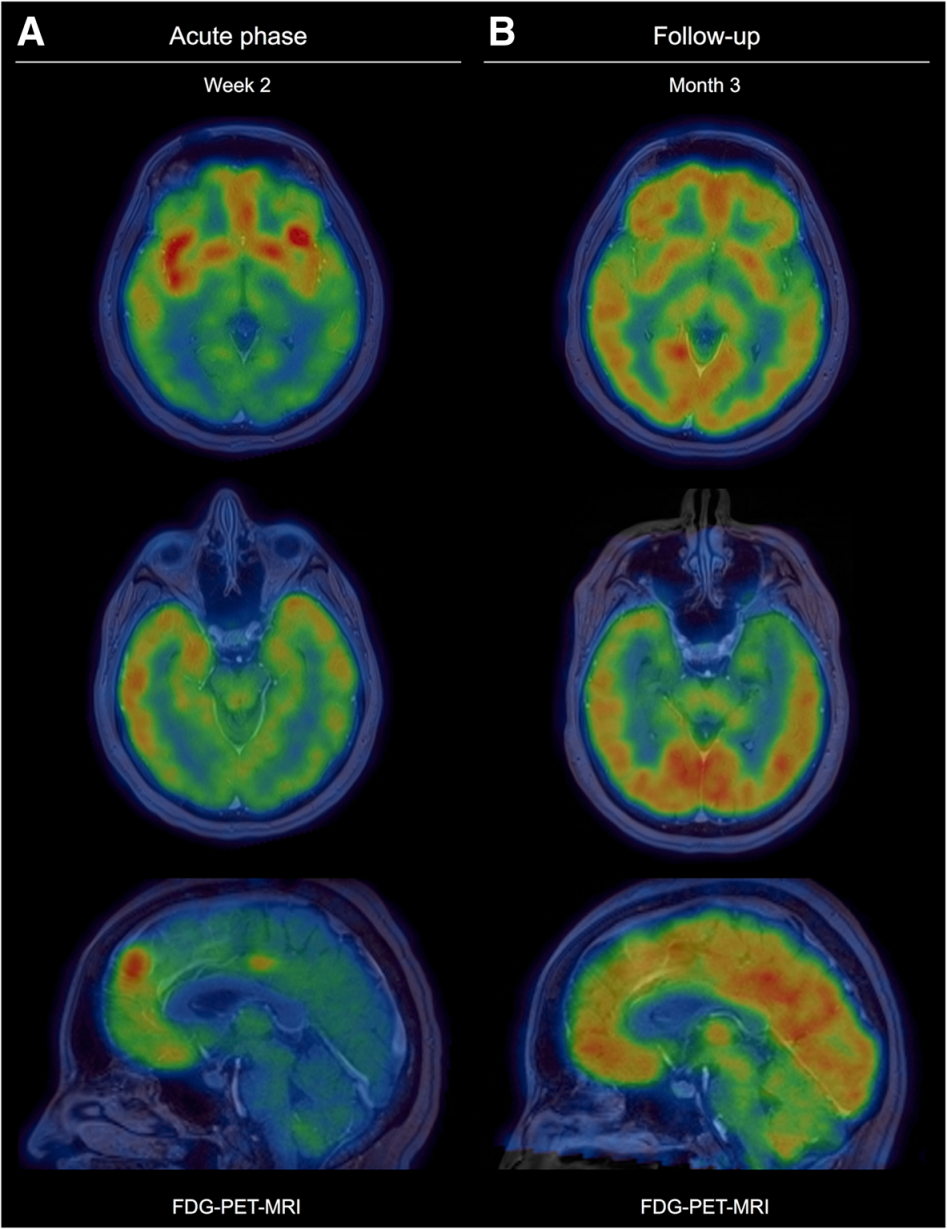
Evolution of glucose metabolism over time. a F^18^-FDG-PET during acute phase of disease (2 weeks after onset) shows bi-insular and bi-frontal glucose hypermetabolism as well as glucose hypometabolism occipital and partially in the parietal lobe. b After 3 months there is a marked bi-insular and bi-hippocampal hypometabolism, whereas prefrontal, occipital and parietal glucose-uptake is normal. F^18^-FDG-PET scans have been co-registered with MRI in order to correlate metabolic pathologies with anatomical structures
